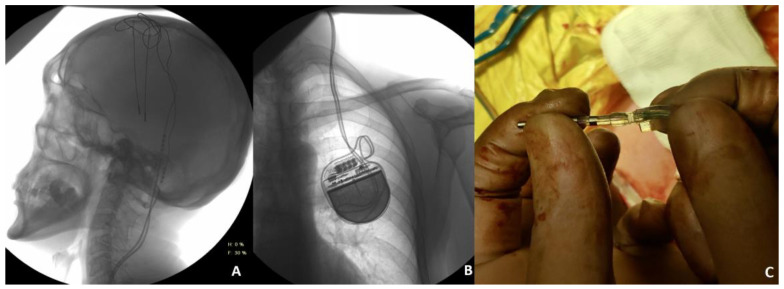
Skull (A) X-ray showing any deformities of the leads. Chest (B) X-ray showing the reversed position of the extensions at the junction connecting to the IPG. Intraoperative view of the lead fracture at the point of connection between extension and IPG (C).
